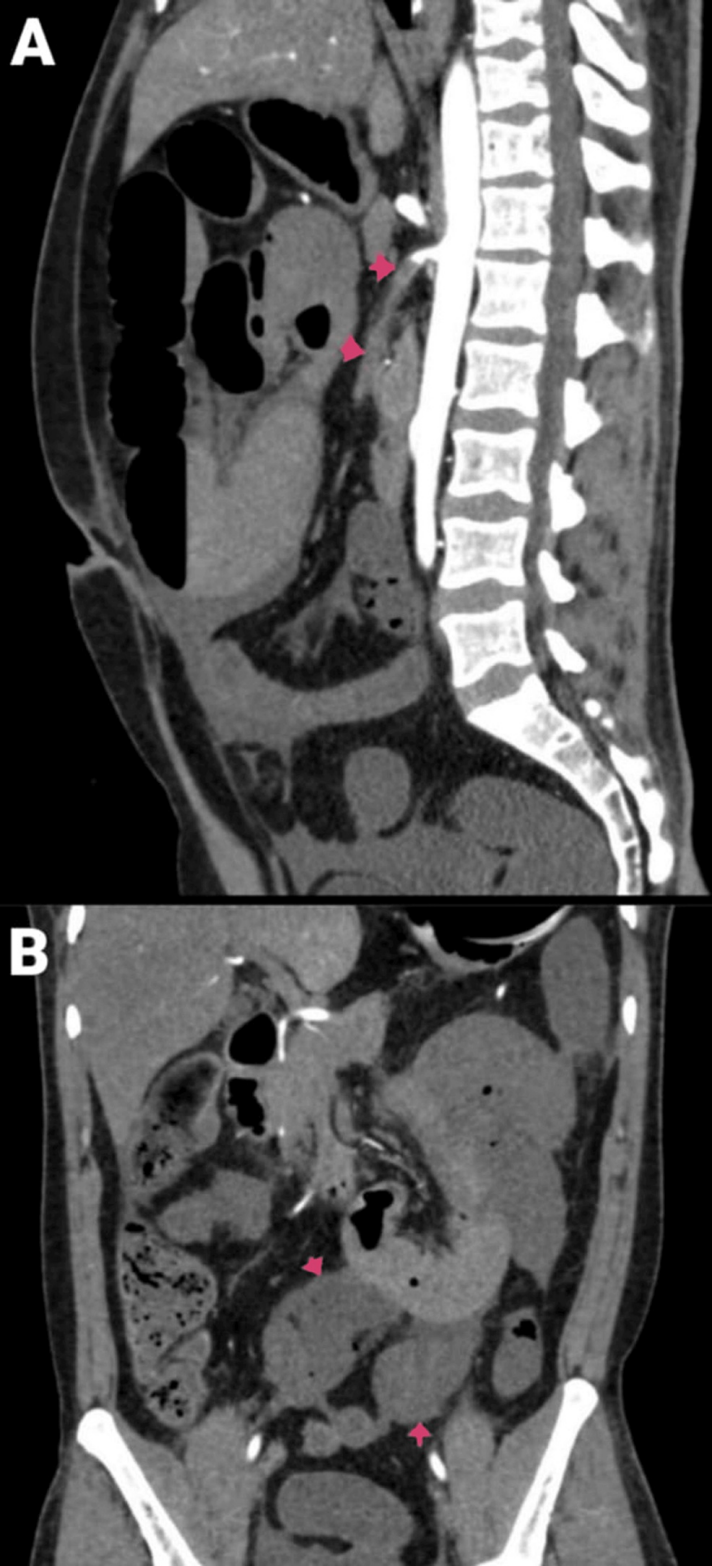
CECT of the abdomen and pelvis with angiography of the patient: (A) sagittal section and (B) coronal section. Arrows are showing SMA thrombosis with dilated jejunal and proximal ileal loops. CECT, contrast-enhanced computed tomography; SMA, superior mesenteric artery
